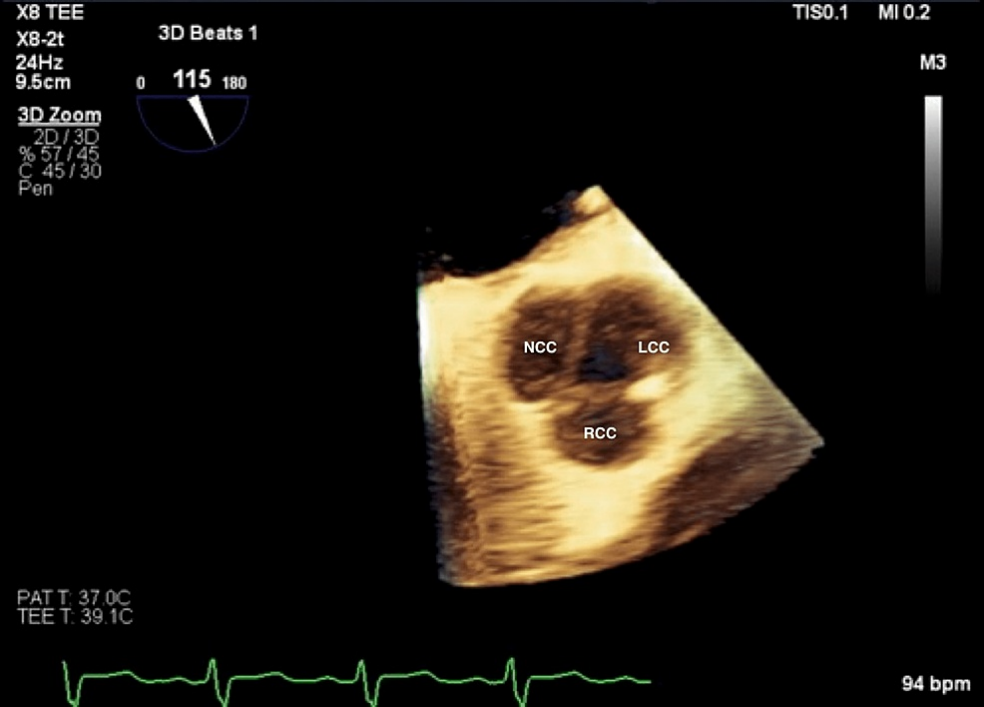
Transesophageal echocardiogram: 3D short axis aortic view at end-diastole The image shows the retraction of the tip of all three leaflets of the aortic valve, particularly the right coronary cusp. NCC - non-coronary cusp; LCC - left coronary cusp; RCC - right coronary cusp
